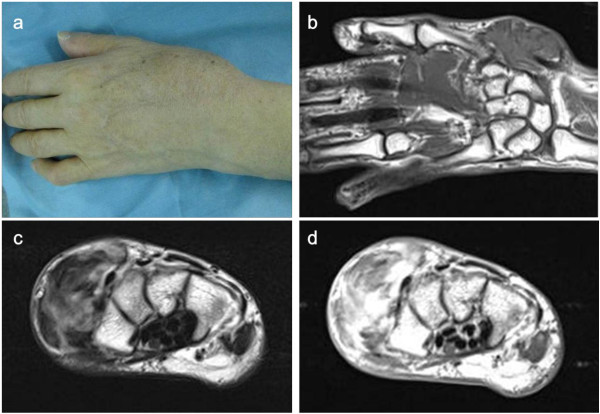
Representative case: case 3. (a) A 62-year-old male patient with synovial sarcoma in the dorsoradial aspect of the hand. (b) Magnetic resonance imaging (MRI) of the tumour showed isointensity on a T1 image, (c) inhomogeneous high on a T2 image and (d) enhancement with the use of gadolinium. The mass spanned the first, second and third compartments of the extensor tendon sheath but with no evidence of bone involvement.
